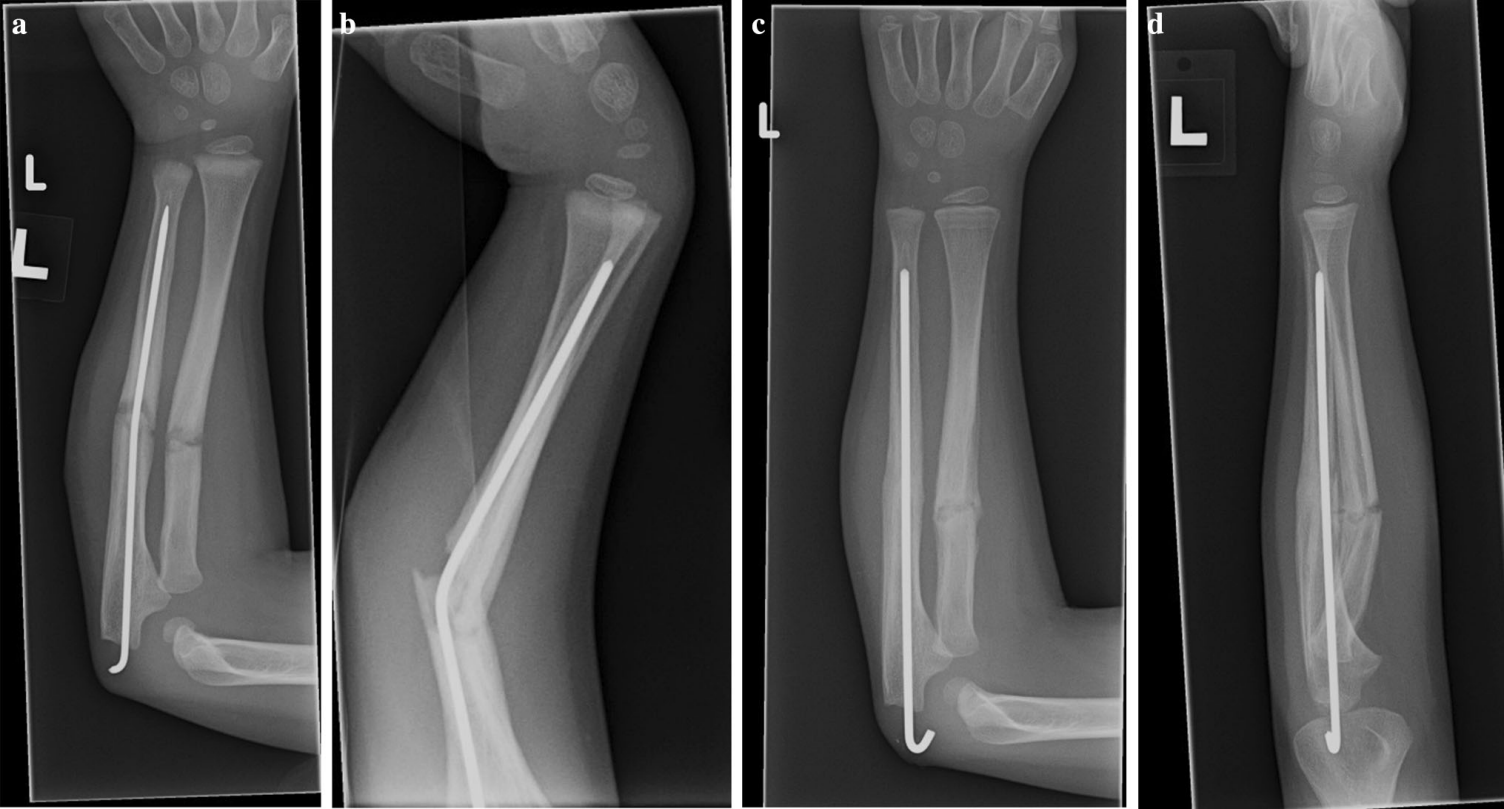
Anteroposterior (a) and lateral (b) radiographs of a 4 year old boy (Patient 3) who sustained a refracture from a fall from bed. He was treated with removal of his ulnar implant and introduction of a new nail (c, d)
